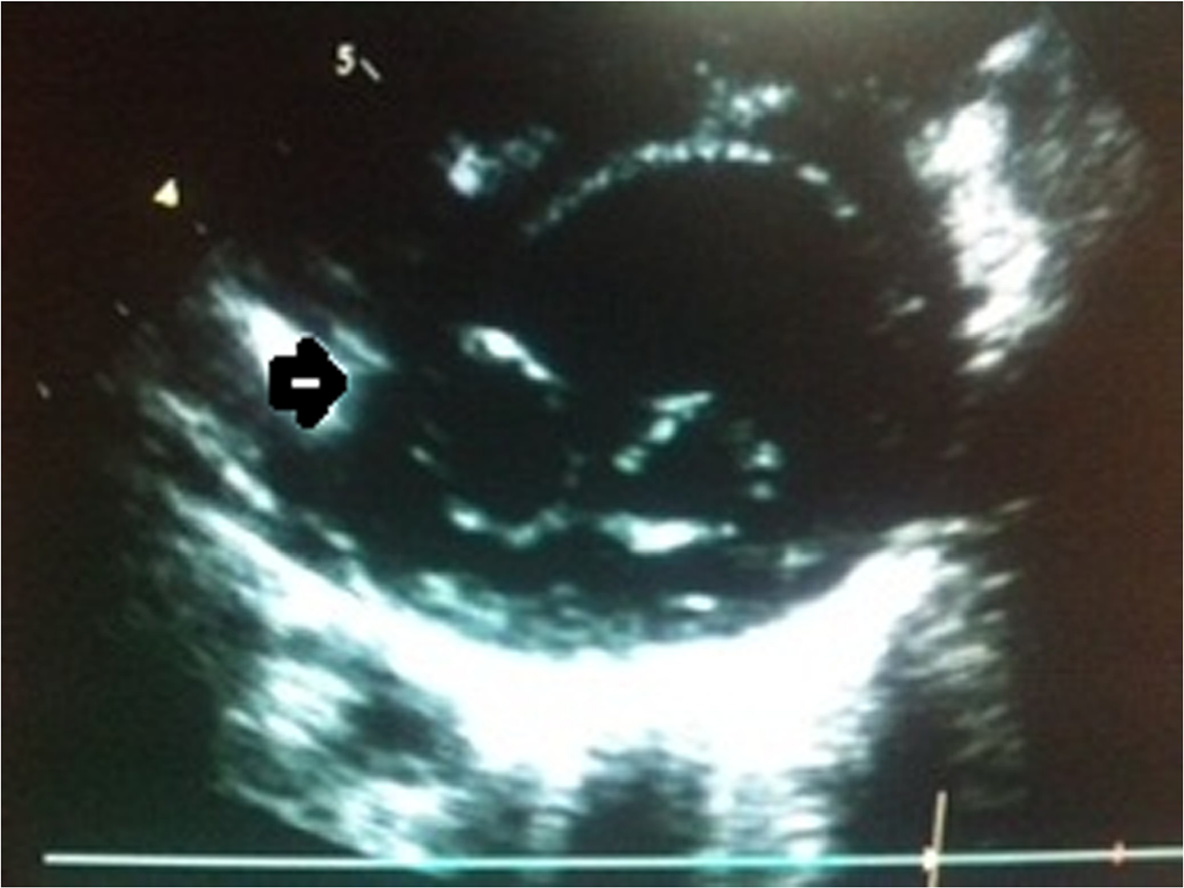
Echocardiography short axis parasternal view showed double orifice mitral valve.
